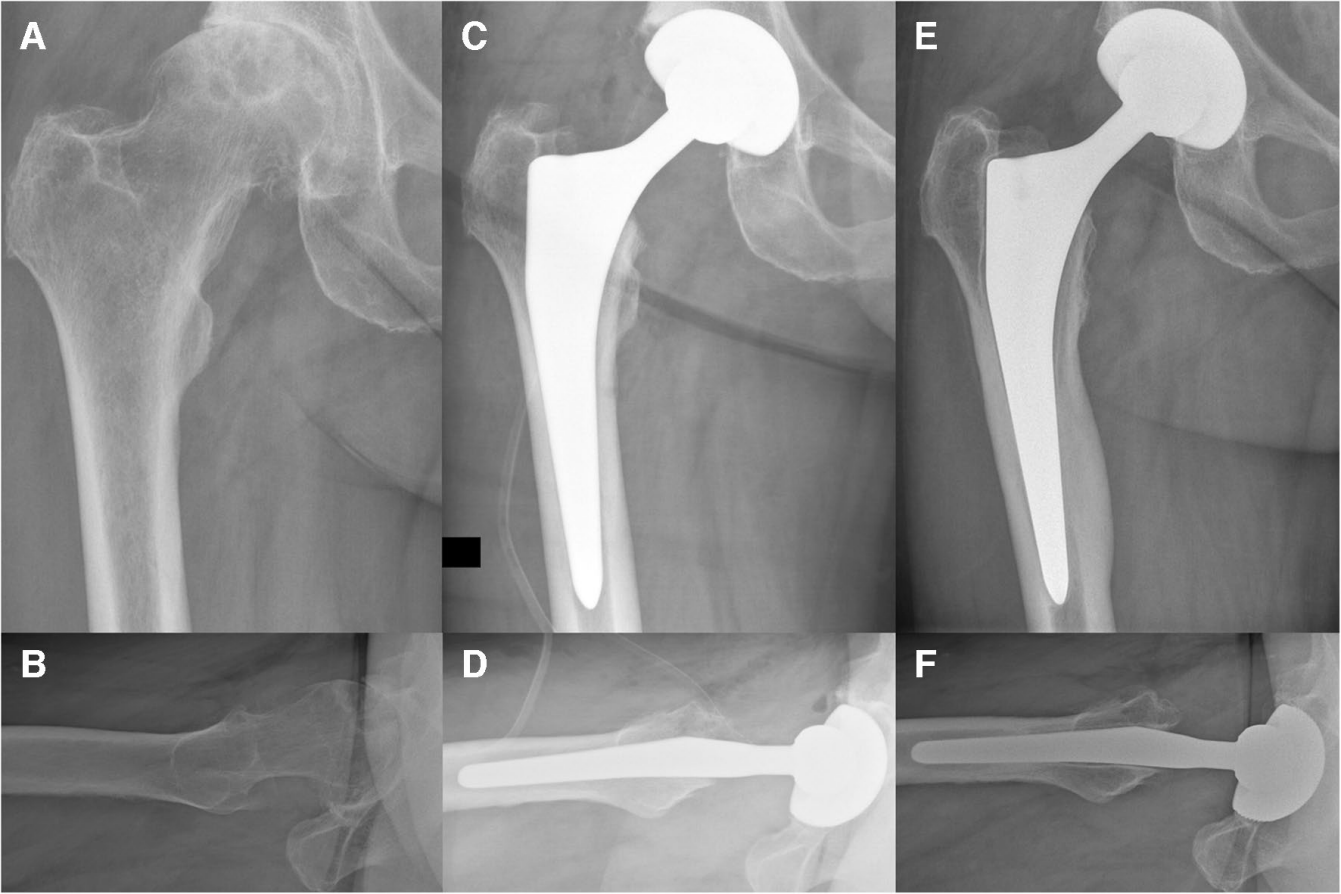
Conventional radiographs of a patient with aseptic femoral component loosening. A, B Preoperative radiographs. C, D The direct postoperative radiographs, whereas E, F show the loose femoral component 3 years and 9 months postoperative
